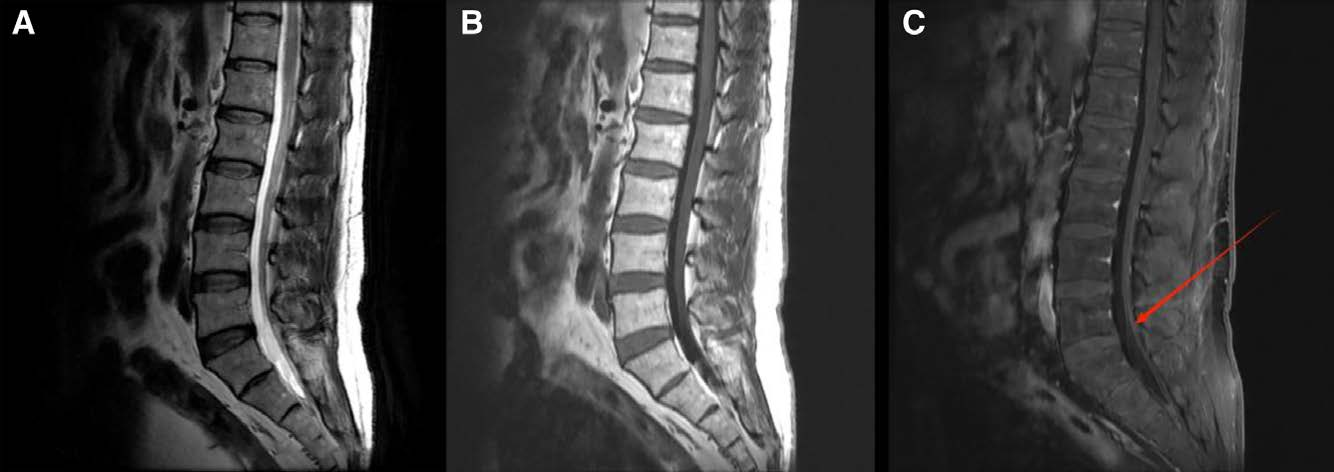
Lumbar MRI. (A) Sagittal T1-weighted imaging (T1WI) of lumbar spine plain scan: no abnormal signals were observed in the conus medullaris and cauda equina nerves; (B) sagittal T2-weighted imaging (T2WI) of lumbar spine plain scan: no abnormal signals were observed in the conus medullaris and cauda equina nerves; (C) sagittal T1WI with fat suppression of lumbar spine enhanced scan: linear enhancement was observed on the surface of the cauda equina nerves; the area pointed by the red arrow is the specific enhanced part. MRI = magnetic resonance imaging.
